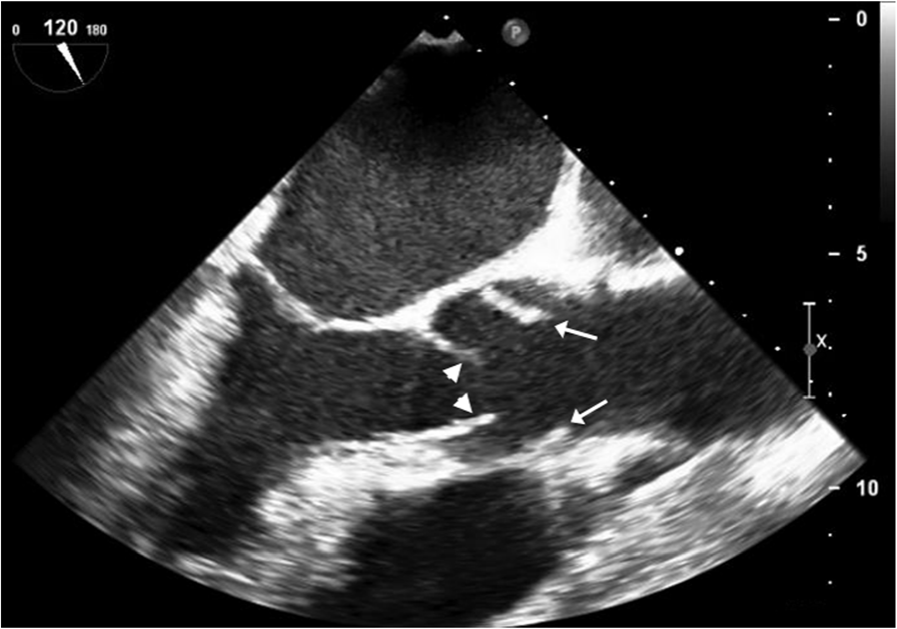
Transesophageal echocardiogram. Transesophageal echocardiographic scan of the aortic root showing the open leaflets of the aortic valve (arrowheads) and the dissection flap just above the valve and within the sinuses of Valsalva (arrows).
